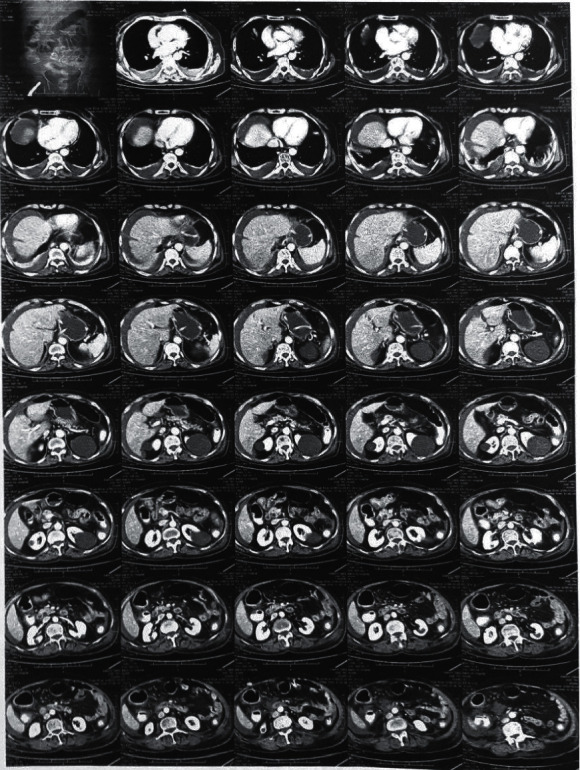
Computed tomography (CT) showing the presence of ascites and pleural effusion.
